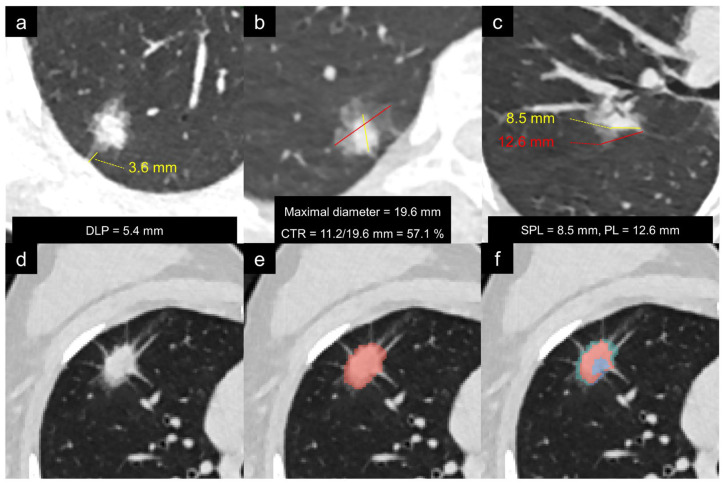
Definitions of radiological features and representative examples of radiomic and habitat-based segmentations. (a) Minimum distance between lesion and pleura (DLP). (b) Consolidation-to-tumor ratio (CTR) based on the maximal diameter of nodule (red line) and solid component (yellow line). (c) The solid component–pleural contact length (SPL, yellow line) and lesion–pleural contact length (PL, red line). (d) Original low-dose CT image. (e) Representative result of the whole lesion radiomic segmentation. (f) Representative result of three habitat segmentations: habitat 1 (green) at the peripheral edge adjacent to the pleura, habitat 2 (pink) in the transitional zone, and habitat 3 (blue) in the central core.
